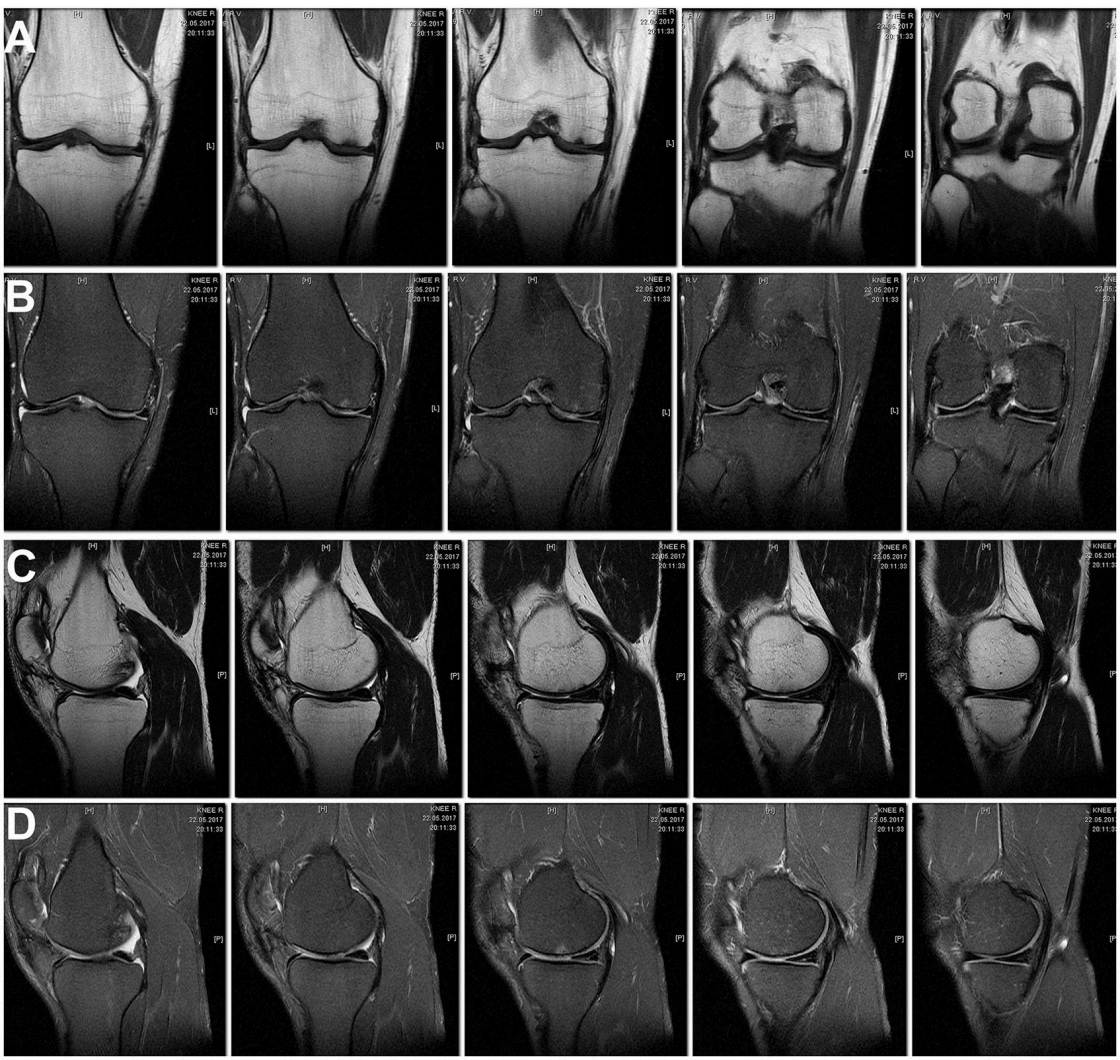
MRI of the right knee 27 months after the surgery. The hyaline cartilage slightly inhomogeneous structure, with restored thickness, in the subchondral compartment an area of osteosclerosis in the form of a small local pathological MR signal. (A) Cor T1FSE; (B) Cor PD fat sat FSE; (C) Sag T1FSE; (D) Sag PD fat sat FSE.
